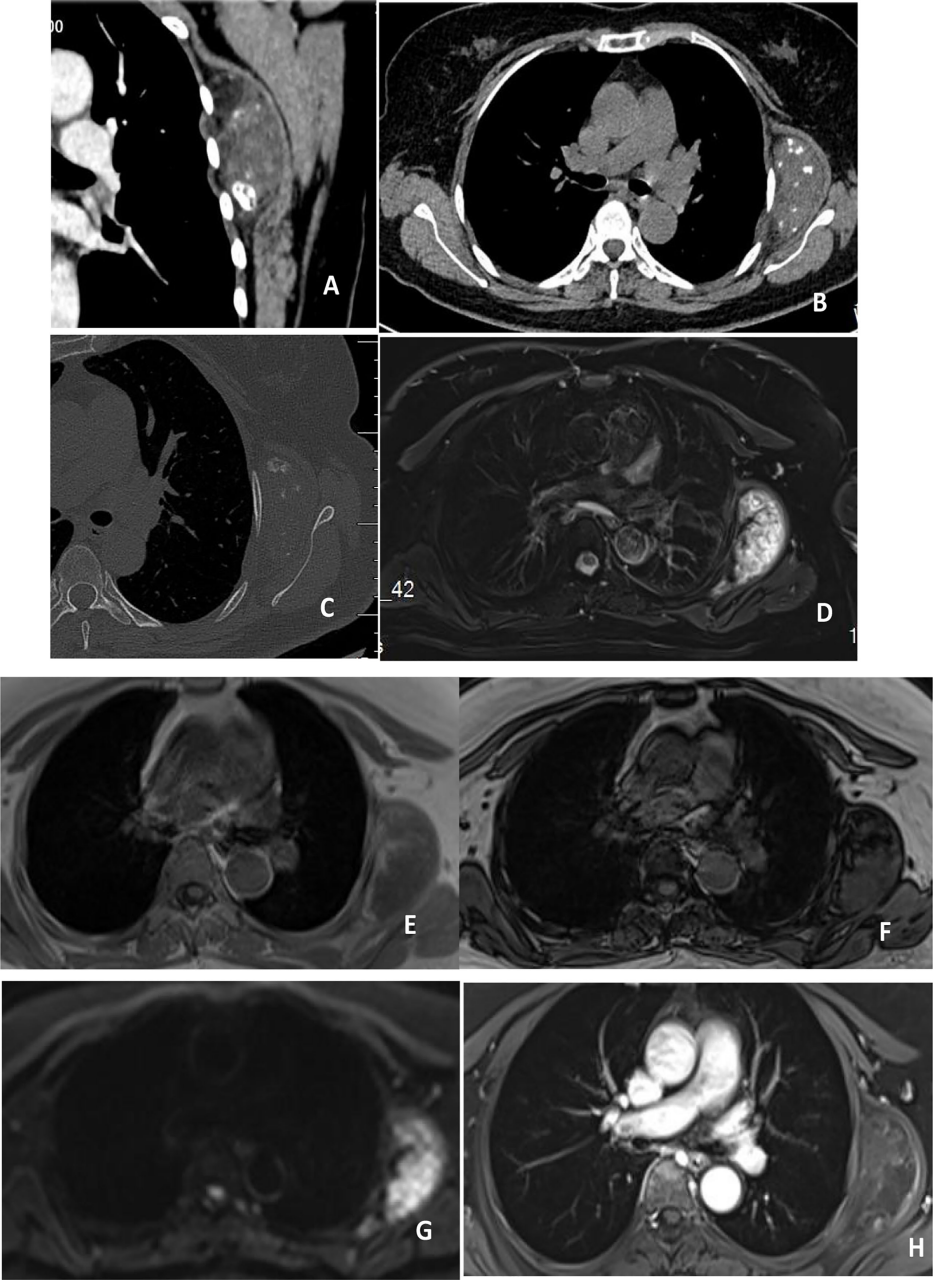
(A–C) showed plain and enhanced CT scan, showing mixed density foci inside the left serratus anterior muscle, calcification and fat density, no significant enhancement after enhancement, and no bone destruction in adjacent ribs. (D–H) showed plain and enhanced MRI, with high signal on T2WI and stripe low signal inside the left serratus anterior muscle, isosignal in T1WI, stripe high signal inside, low signal in reverse phase, and high signal in DWI. After enhancement, no significant enhancement was observed in the center of the lesion, and enhancement was observed at the edge.
